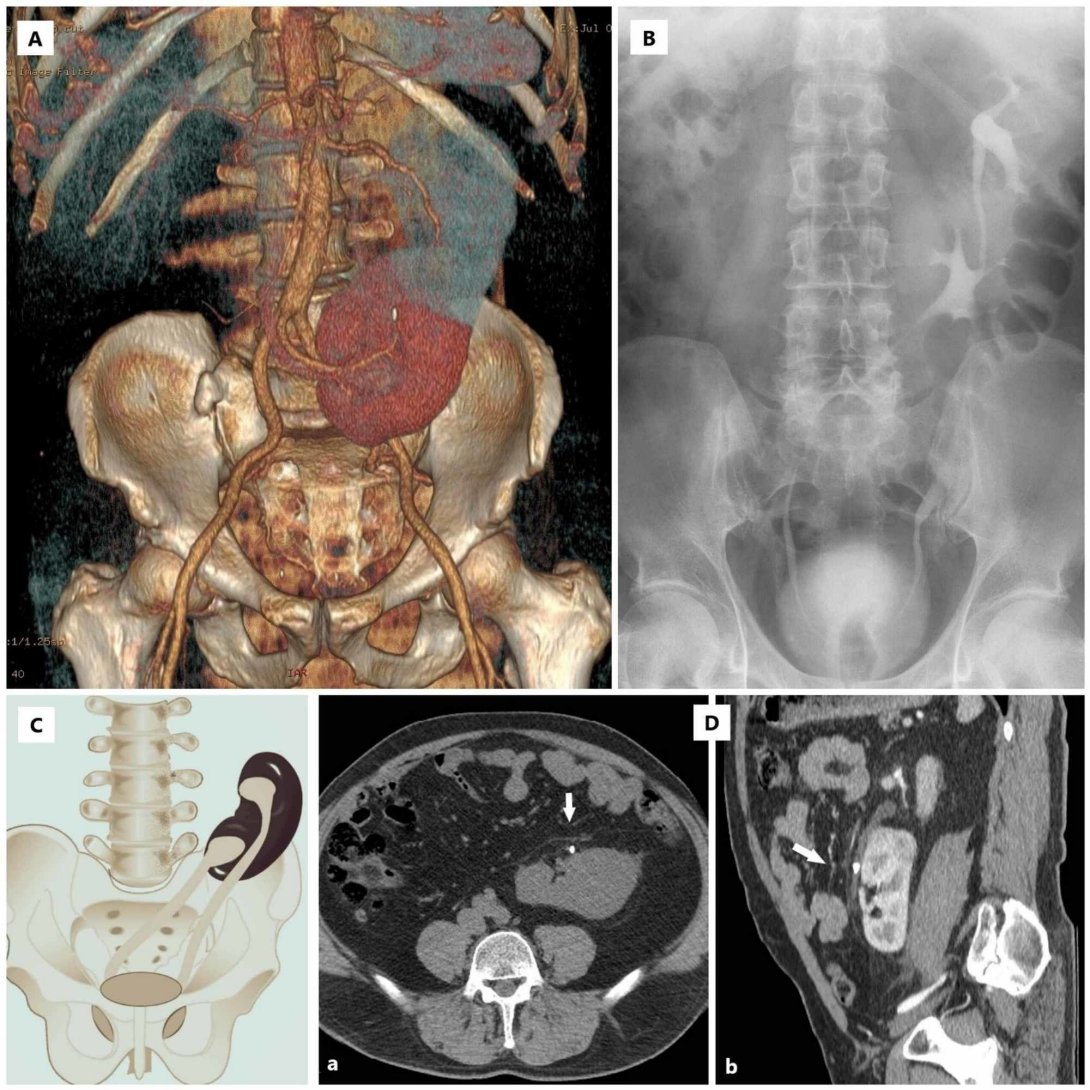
Radiological features of present case (A) 3D computed tomography revealed inferior right-to-left crossed fused renal ectopia with the abnormal arterial supply and stone in the projection of proximal ureter of the upper kidney; (B) Postoperative IVU showed no stone shadows or signs of obstruction. The ureter of ectopic kidney crosses the midline and enters into the bladder at the normal position; (C) Abnormal anatomy of the urinary tract in the present case (scheme); (D) Preoperative abdominal CT scans in axial (a) and sagittal (b) plane revealed stone (arrow) in the proximal part of the ureter of the upper kidney. IVU = intravenous urography, CT = computed tomography.
